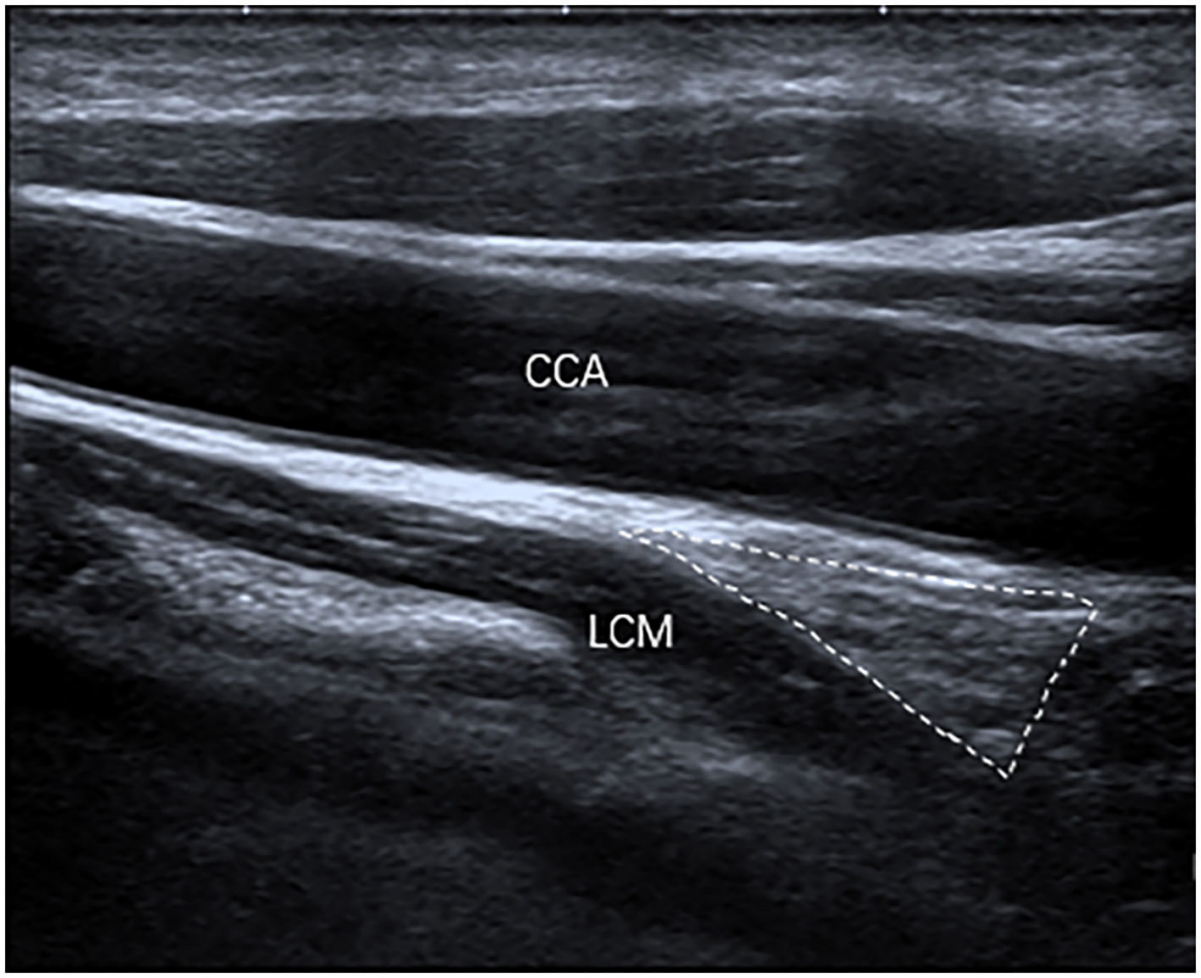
The SG, with a triangular shape (outlined) and hyperechogenicity, was located between the common carotid artery (CCA) and the longus colli muscle (LCM) on the longitudinal ultrasound image.
